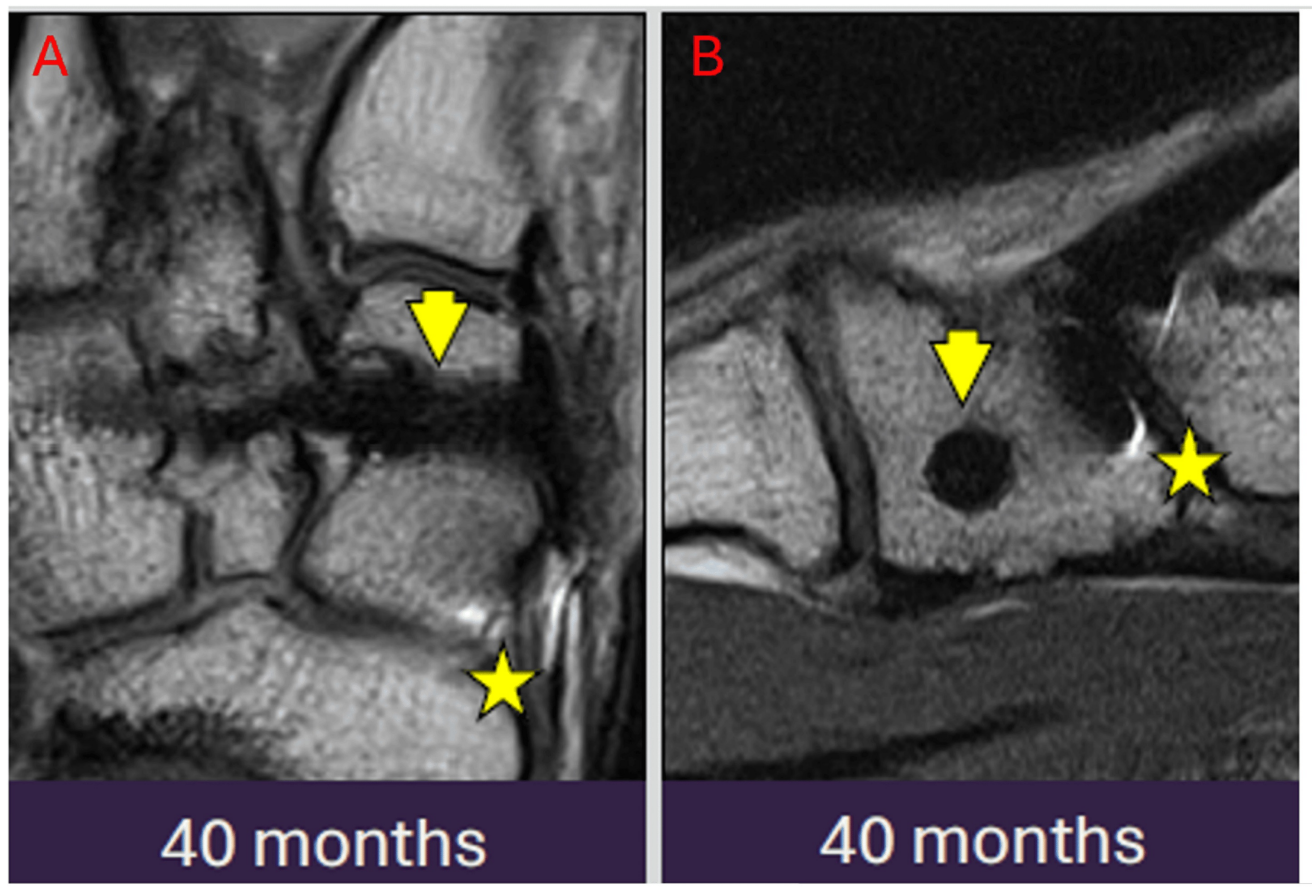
Forty months post-operative magnetic resonance imaging showing more artifacts from the titanium implant (star) as compared to the remnants of the magnesium implant which appear hypointense (arrowhead). The images show an axial cut (A) and a sagittal cut (B).
